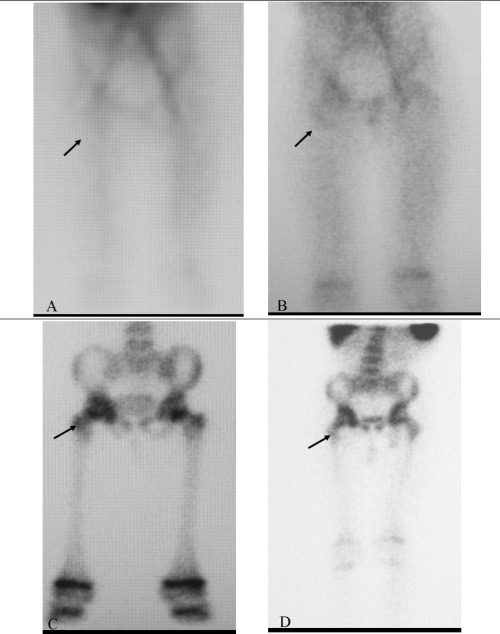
5 year old female patient with suspected right hip infection.Figure 1A, Figure 1B, Figure 1C. Three-phase bone scintigraphy shows positiveuptake in the region of right hip (arrow). Figure 1D. ^99m^Tc-HMPAOlabeled WBC scintigraphy shows positive uptake in the same region (arrow)compatible with osteomyelitis. Result: Infected right hip
